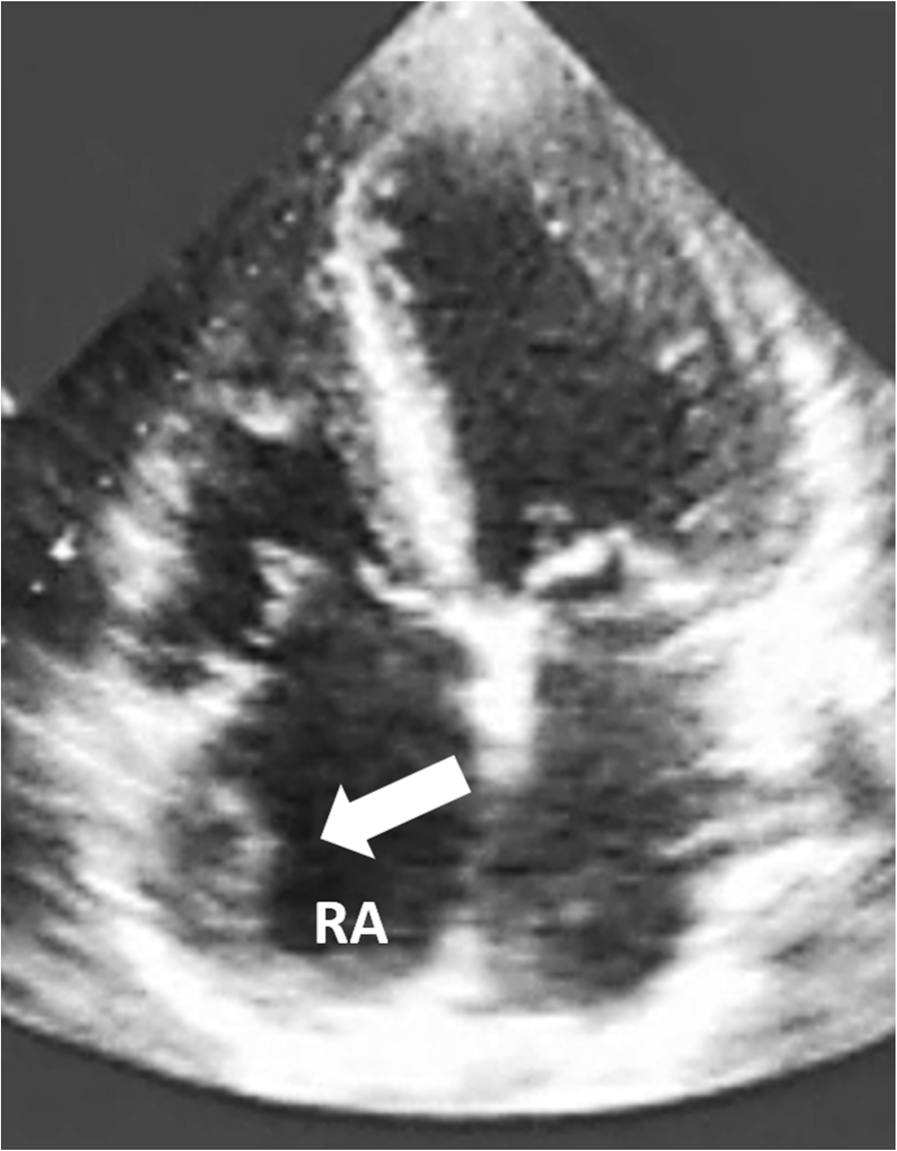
On transthoracic echocardiogram, one irregular and heterogeneous mass (5.6cm^2^) over the lateral wall of the right atrium (RA) (*white arrow*)
